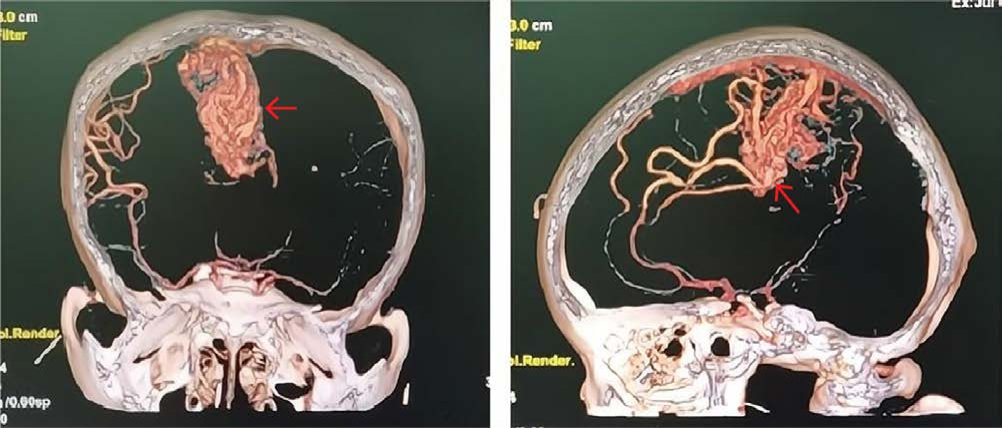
CTA of patient’s brain shows the lesion of AVM (arrow). AVM = arteriovenous malformation.
